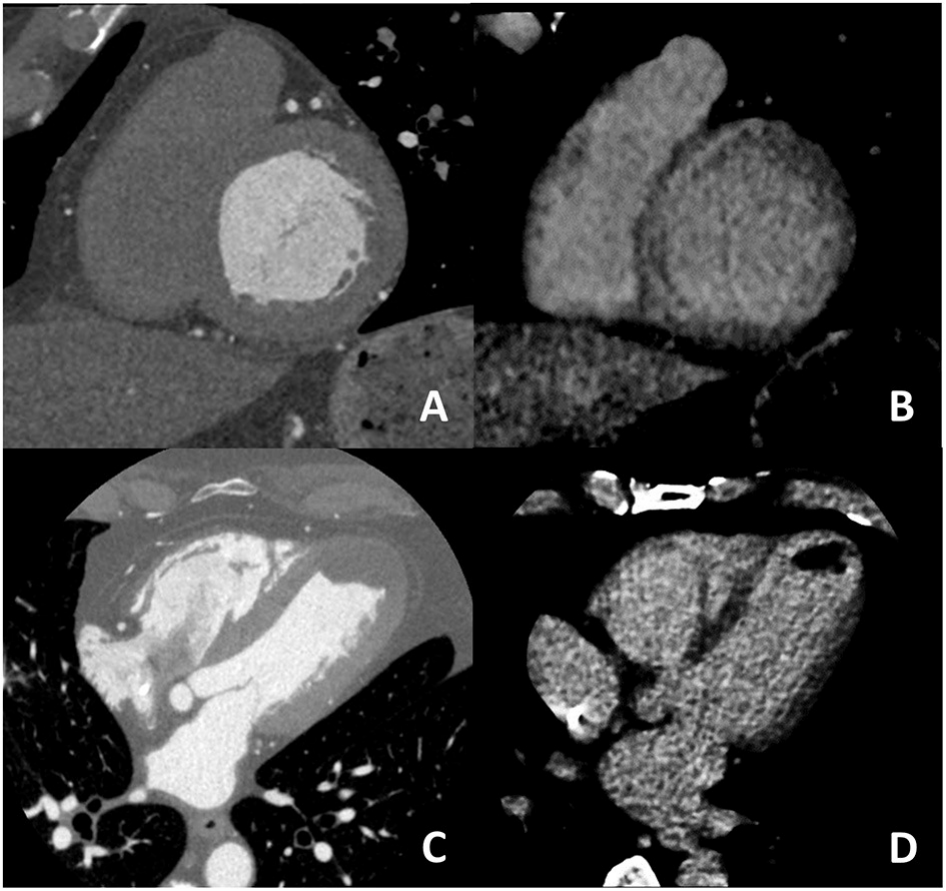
In (A,B) an example of non-ischemic fibrosis on the interventricular septum is shown; more specifically, in (A) angiographic phase CT is presented with corresponding late CT acquisition well demonstrating non-ischemic mid-wall myocardial fibrosis in (B). In (C,D) a case of ischemic cardiomyopathy is presented; an apical left ventricular thrombus is evident both at first pass CT (C) and at late phase CT (D) from which the ischemic subendocardial fibrosis could be evidenced.
